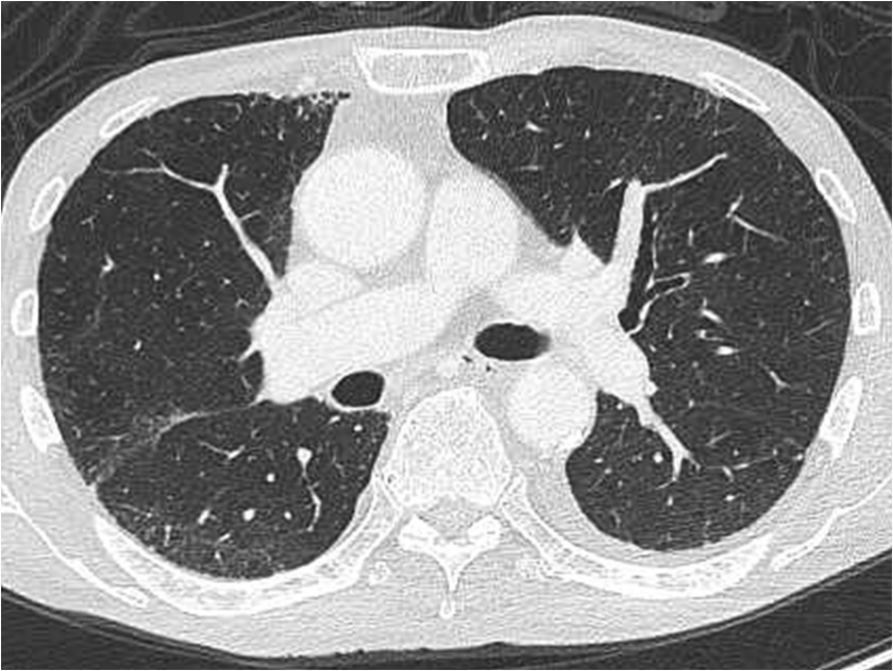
Chest computed tomography at 4 weeks after starting treatment. The ground-glass opacity in both lungs disappeared after discontinuation of gemcitabine and initiation of corticosteroids and pulmonary support.
